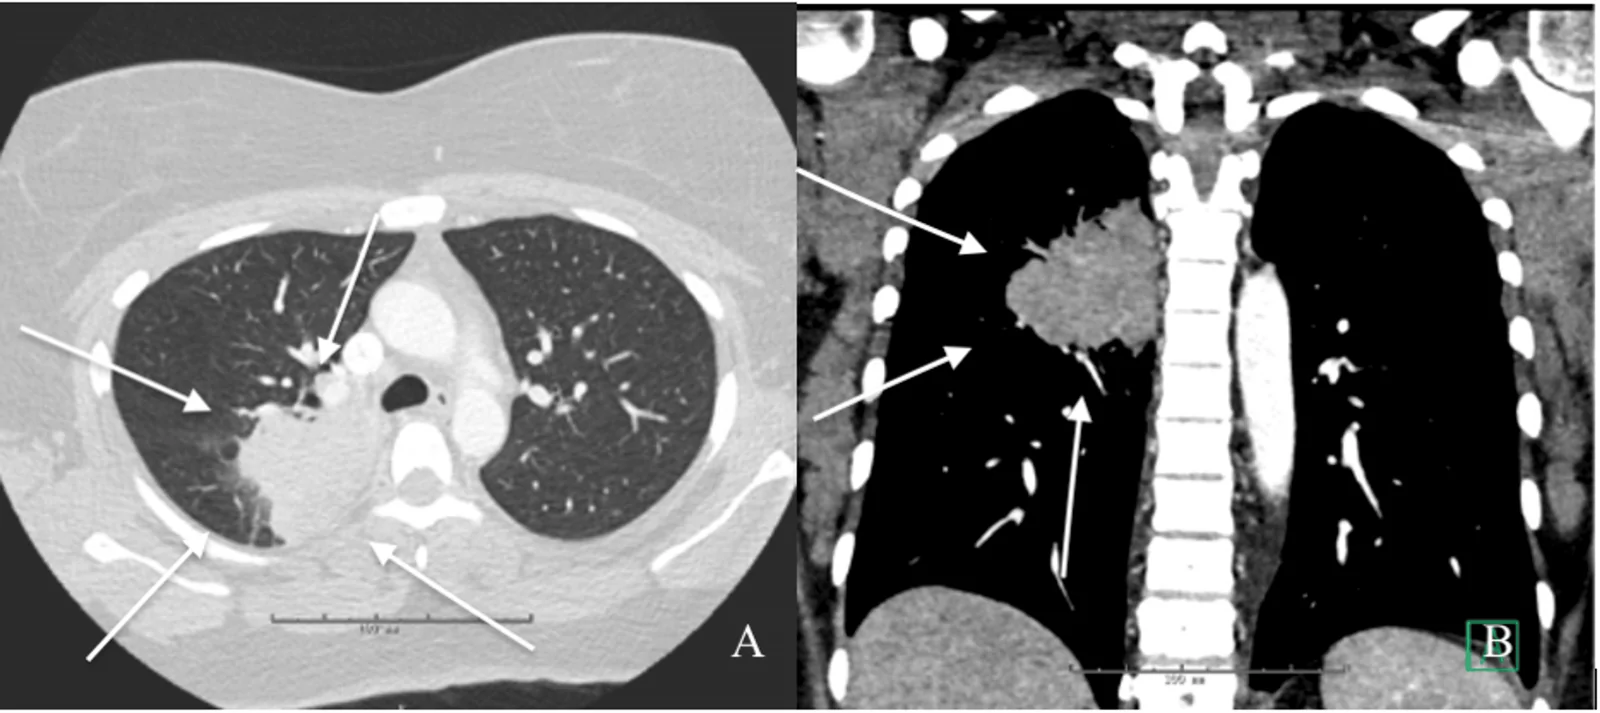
Initial contrast-enhanced CT chest imaging. A: axial view; B: coronal view demonstrating a heterogeneous right upper lobe pleural-based mass-like consolidation abutting the right hilum with adjacent centrilobular nodularity concerning an infectious etiology. Arrows point to a heterogeneous right upper lobe mass abutting the pleura and right hilum.
